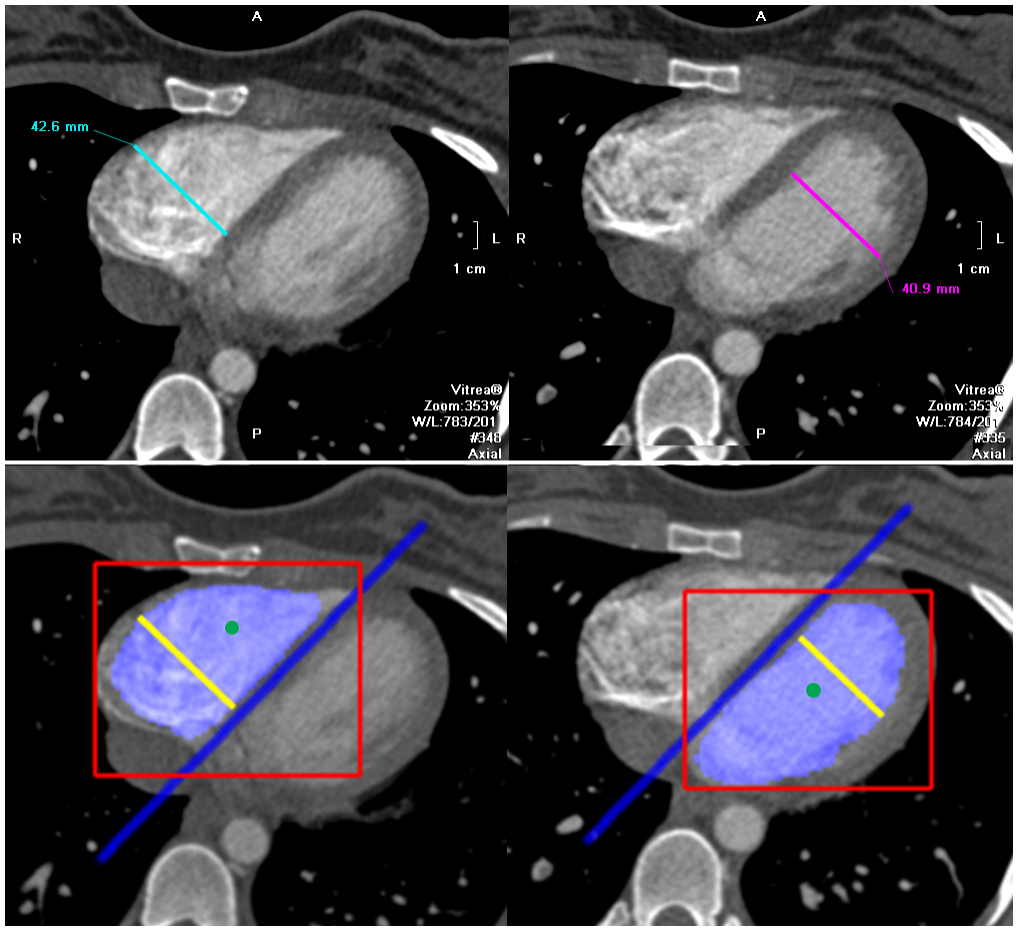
Axial images of a computed tomography pulmonary angiography of a 27 years old female with acute pulmonary embolism. Top row: manually estimated axial ventricular diameters. Bottom row: automatically estimated axial ventricular diameters and depiction of the different steps followed to compute them: ventricle detection (red boxes), seed positioning (green dots), interventricular septum estimation (blue line), ventricle segmentation (blue areas) and ventricular diameter estimation (yellow lines).
